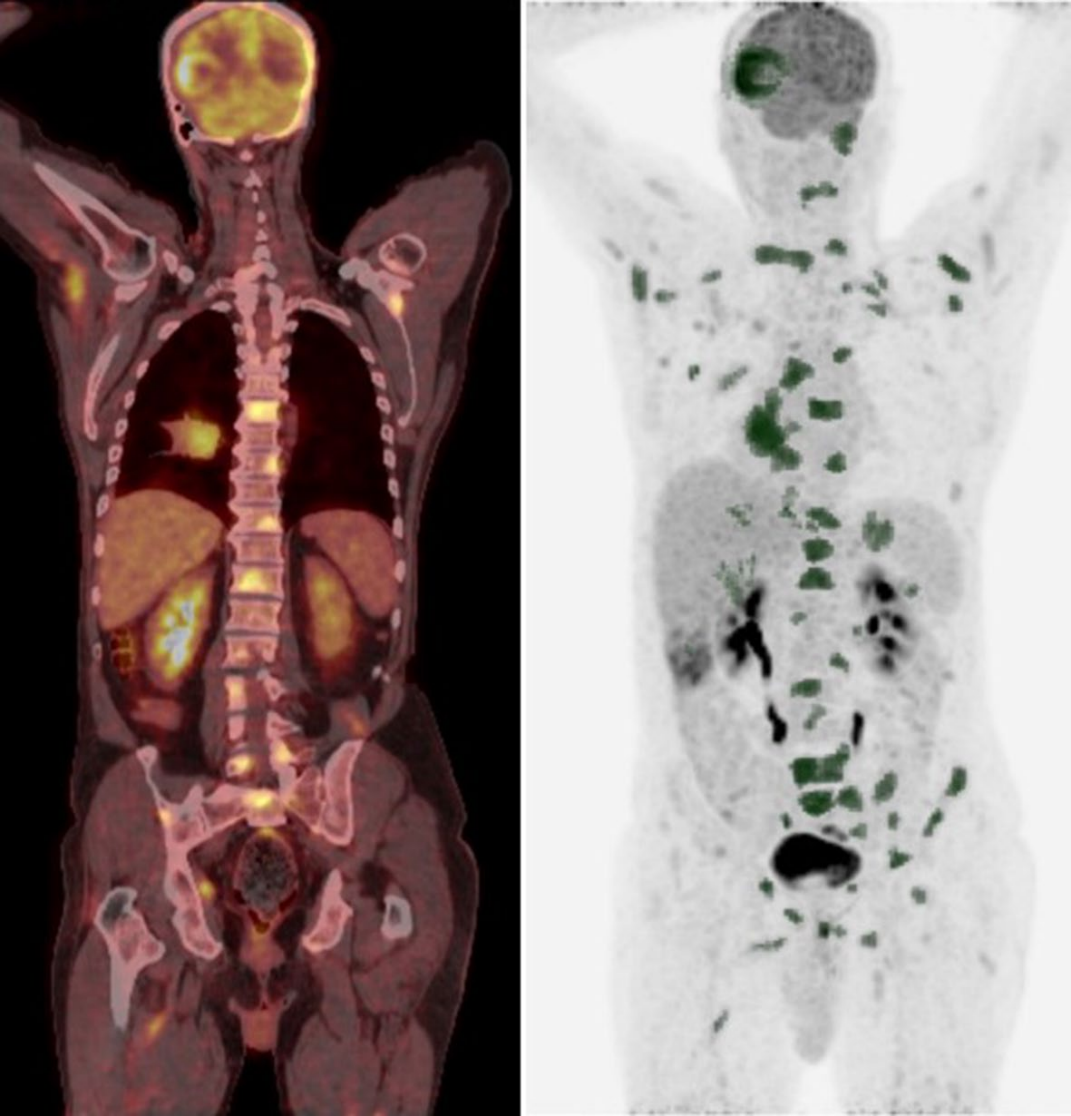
Representative ^18^F-FDG PET/CT MIP and coronal images for a patient with a high whole-body tumor burden (WBTB). A 61-year- old male patient with pathologically proven right lower lobe NSCLC, adenocarcinoma. FDG uptake was abnormally increased at the primary tumor mass as well as multiple metastatic nodal, osseous brain, and muscular deposits, corresponding SUVmax, SUVpeak, SUVmean, tu MTV, tu TLG, WB MTV, and WB TLG 4.9, 4.2, 2.9, 33, 96.03, 317.53, 931.88, respectively
